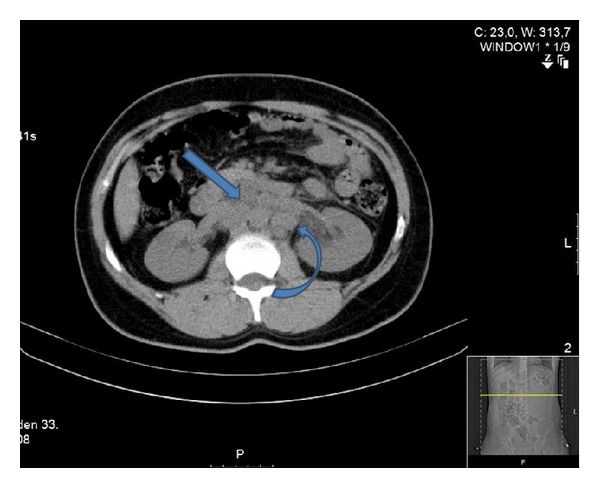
Para-aortic extensive lymphadenopathy in abdominal CT.
